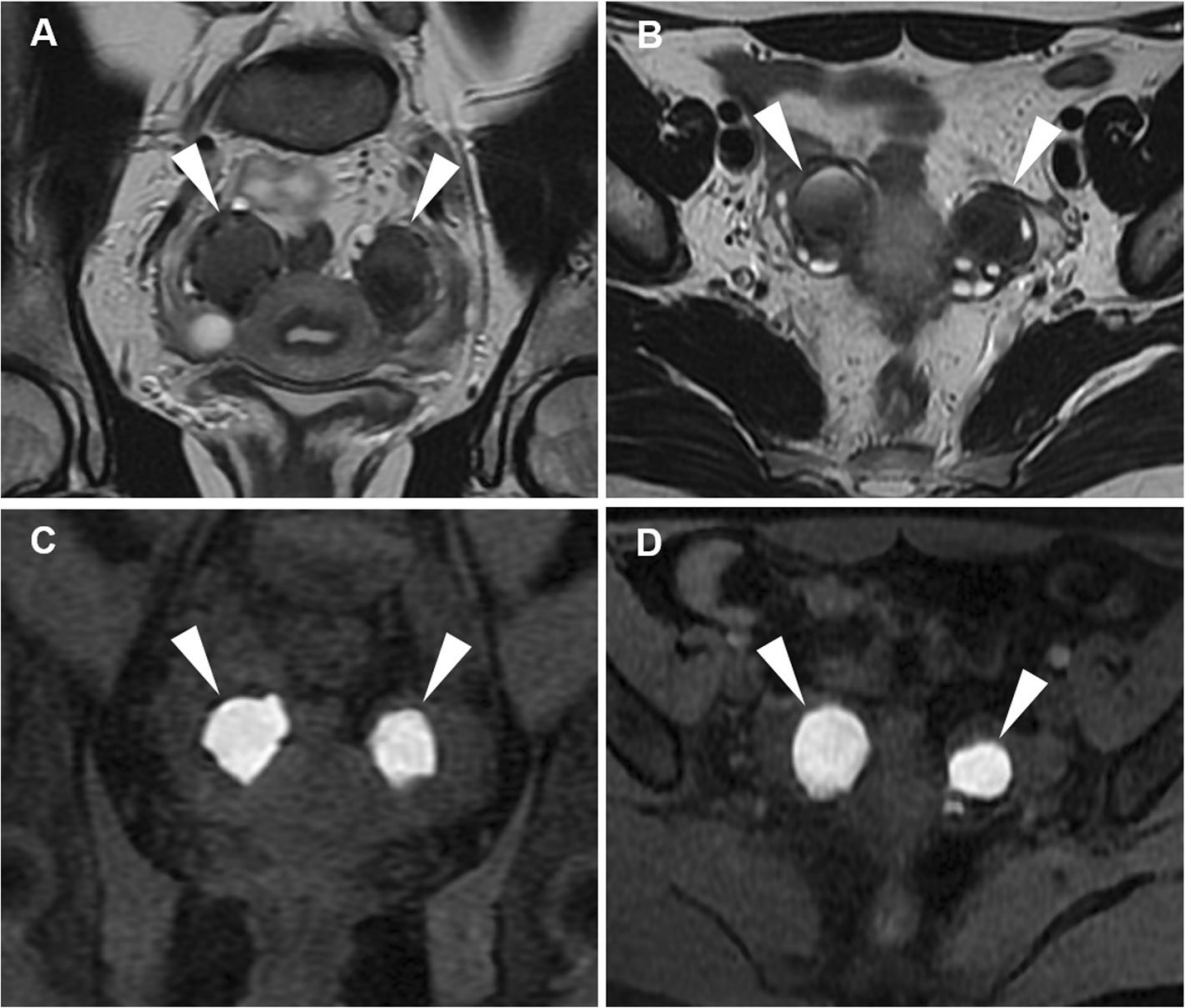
MRI of bilateral endometriomas in a 31-year-old woman suffering from lumbar and pelvic pain. Oblique-axial (a) and oblique-coronal (b) T2-weighted images show bilateral endometriomas (arrowheads) with variable grade of low signal intensity until complete signal loss (‘shading sign’). On oblique-axial (c) and oblique-coronal (d) precontrast fat-suppressed T1-weighted images, the cysts display homogeneous high signal intensity (arrowheads)
